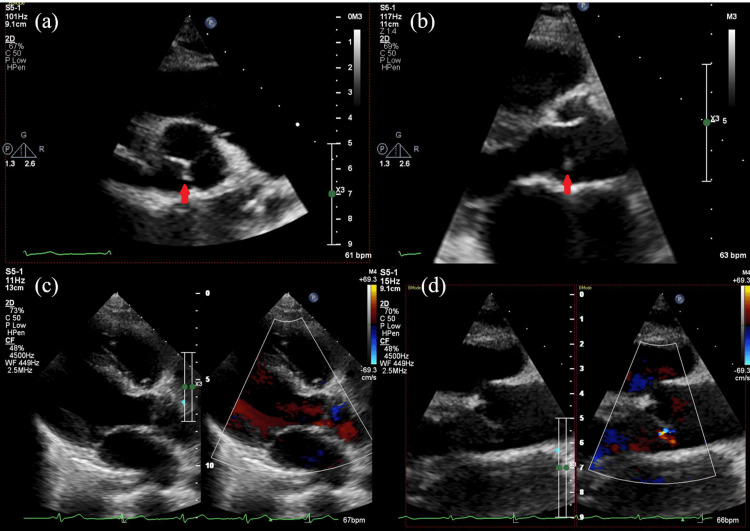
Transthoracic echocardiography at the beginning of treatment revealing vegetation on the aortic valve (red arrow) (a) (b) and mild aortic regurgitation (c) (d)
